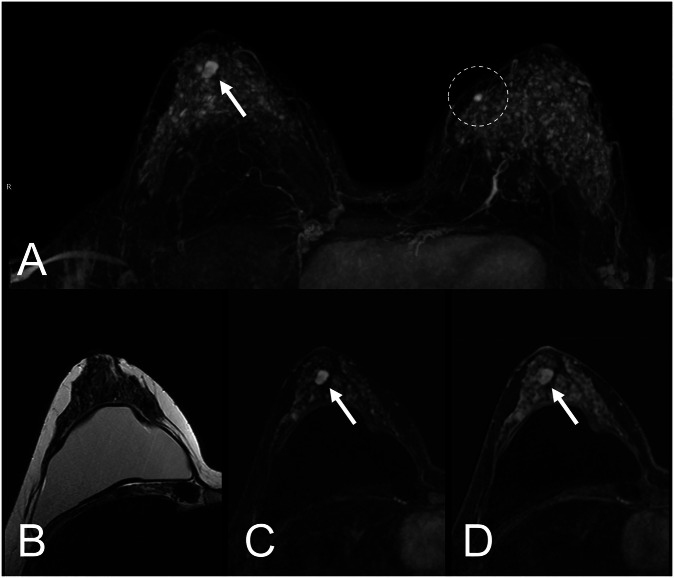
42-year old patient with dense breasts (ACR D) and marked bilateral BPE. The lesion (arrow) is visible on the MIP (A) and T2w (B) as well as early (C) and late (D) contrast-enhanced subtractions. The lesion is located in ROI 5, and it presents as an ill-defined mass with strong initial enhancement and heterogeneous wash-out, corresponding to a Kaiser score of 8 (highly suspicious). Targeted ultrasound-guided biopsy revealed invasive breast cancer NST G3. Note that a small benign lesion < 5 mm is visible also on the MIP (dashed circle in A)
